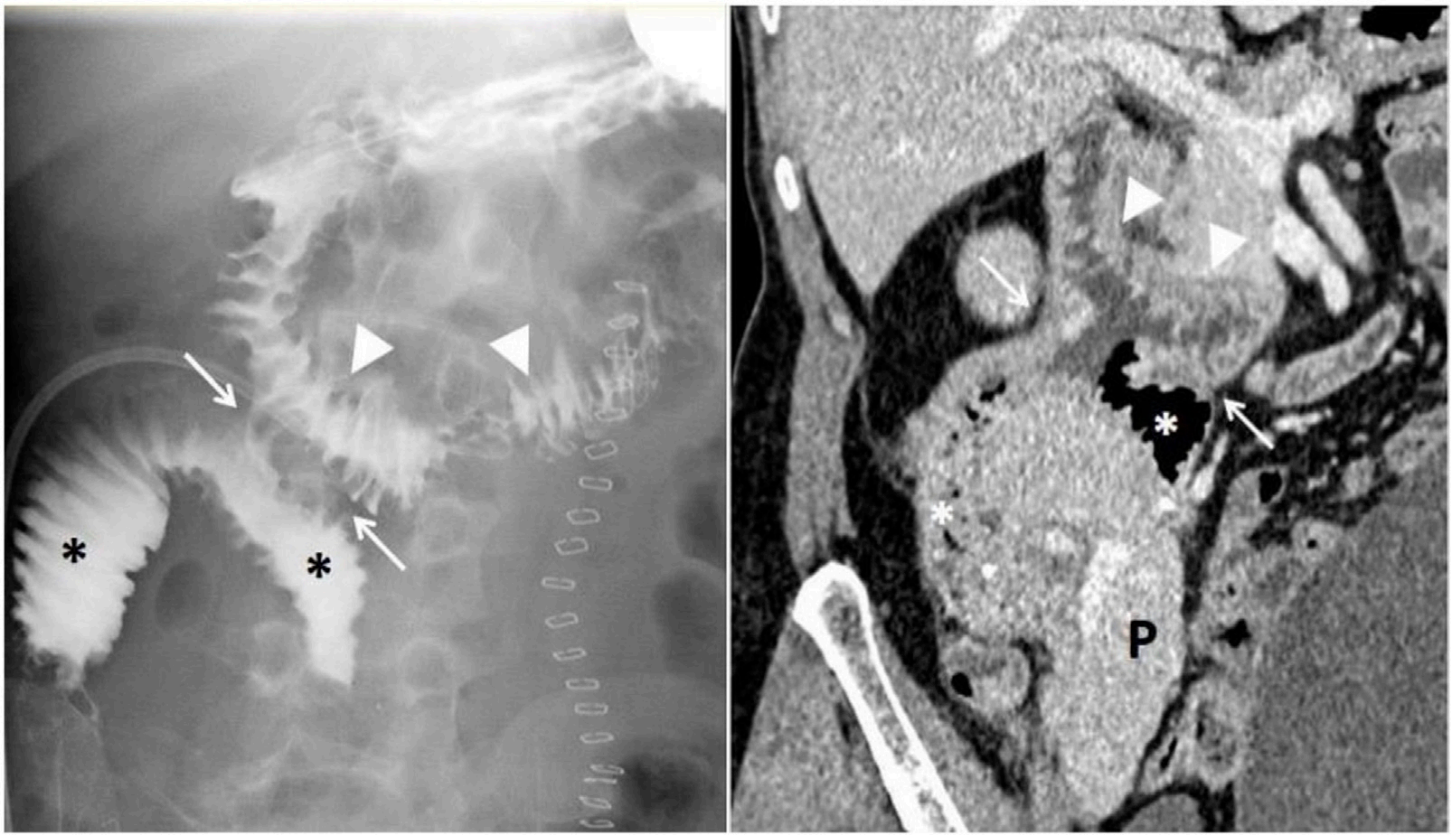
Pancreas transplantation with retrocolic graft placement and duodenoduodenostomy for exocrine drainage. Esophagogastroduodenal transit study with Gastrografin (right) demonstrates proper contrast passage through the recipient’s duodenum (arrowheads), the side-to-side duodenoduodenal anastomosis (white arrows), and the graft’s duodenal segment (asterisks), with no evidence of leakage. Abdominal contrast-enhanced computed tomography of the same patient (left) shows a normoenhancing pancreatic graft (P), without collections or other complications.
